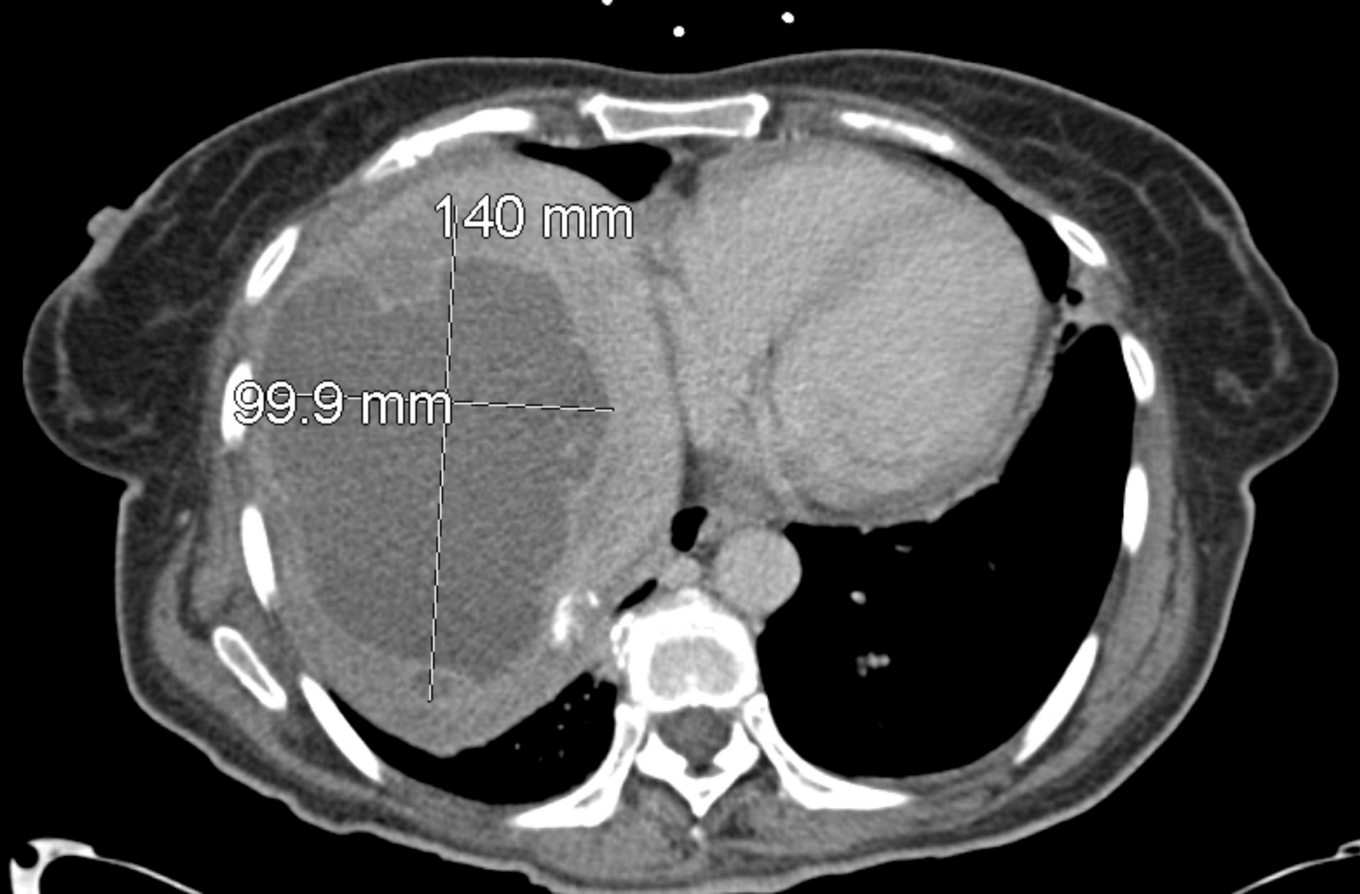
CT abdomen and pelvis revealing a 14 x 10 x 13 cm heterogeneous mass localized within the right hepatic lobe
